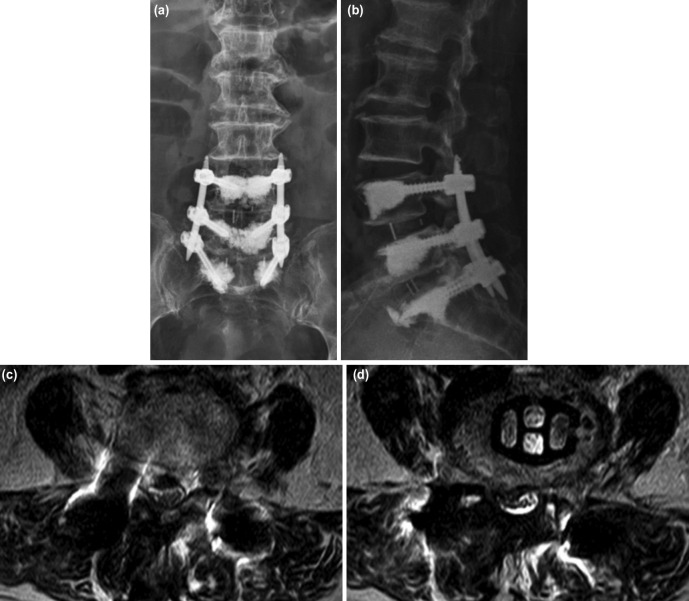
Postoperative radiograph and MRI image at the second surgery (ALIF). (a) AP, (b) lateral, (c) L4 posterior arch level, (d) an at L4-L5 level.
